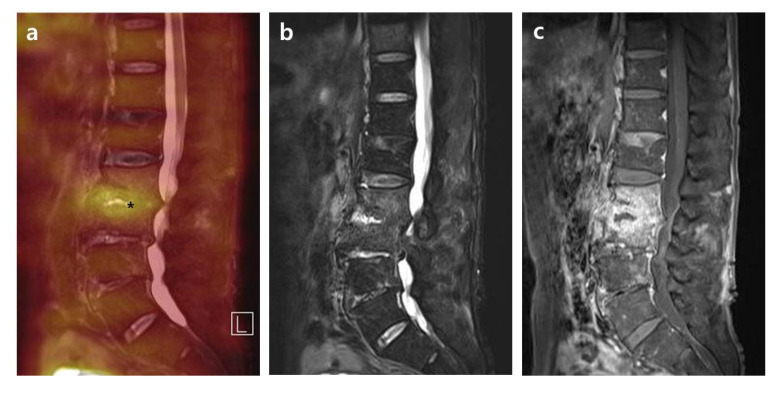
Simultaneous FDG-PET/MRI with the location of SUV_max_ on the intervertebral structure after successful antimicrobial therapy. A 78-year-old male patient shows PVO of L2–3 on simultaneous FDG-PET/MRI with SUV_max_ 3.17 on the intervertebral structure (asterisk) after 30 days of vancomycin (CRP 1.70 mg/dL). In the distribution pattern of the PVO lesion, FDG uptake on FDG-PET (a) and high signal intensity on T2FS (b) are limited on the destroyed disc and endplates rather than the bone and soft tissue. However, T1C shows significantly increased enhancements on the overall PVO lesion (c). FDG-PET/MRI, ^18^F-fluorodeoxyglucose positron emission tomography/magnetic resonance imaging; SUV_max_, maximum standardized ^18^F-fluorodeoxyglucose uptake value; PVO, pyogenic vertebral osteomyelitis; CRP, C-reactive protein; T2FS, T2-weighted fat saturation magnetic resonance imaging; TIC, T1-weighted contrast magnetic resonance imaging.
